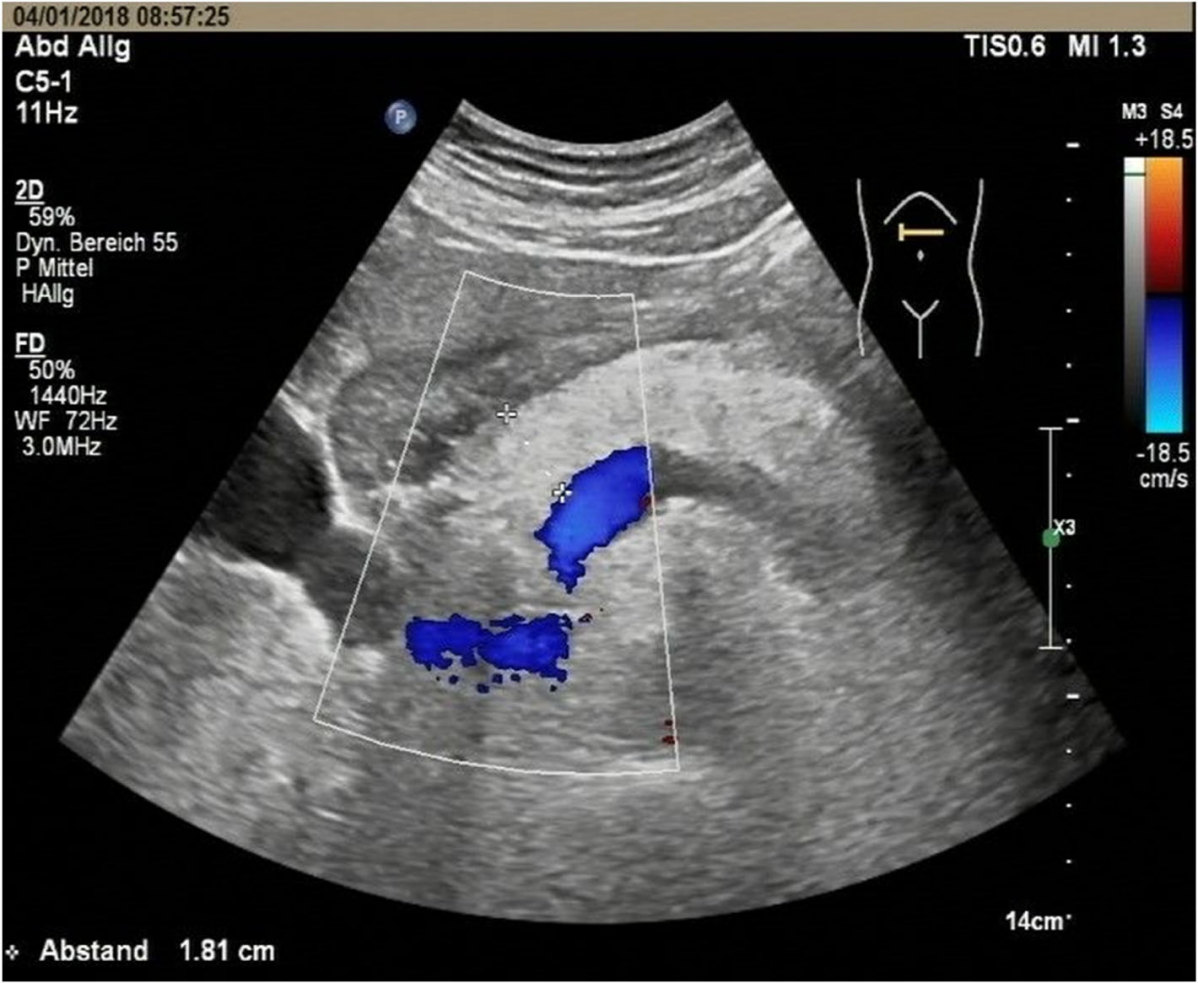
Ultrasound of the patient demonstrating prominent edematous pancreas
